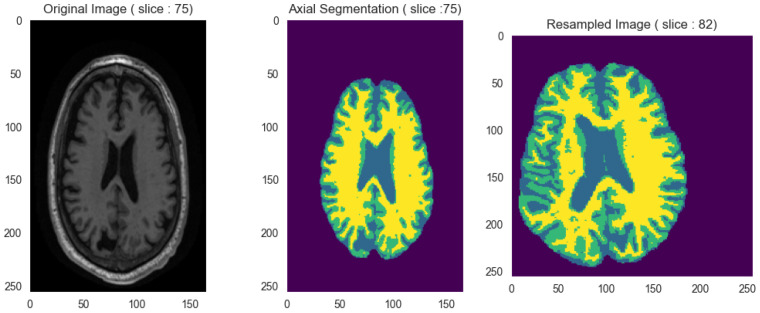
Spatial normalization and resampling process on axial section. From left to right: (1) Original MRI image with variable dimensions. (2) Tissue segmented image. (3) Registered and resampled image in MNI space.
